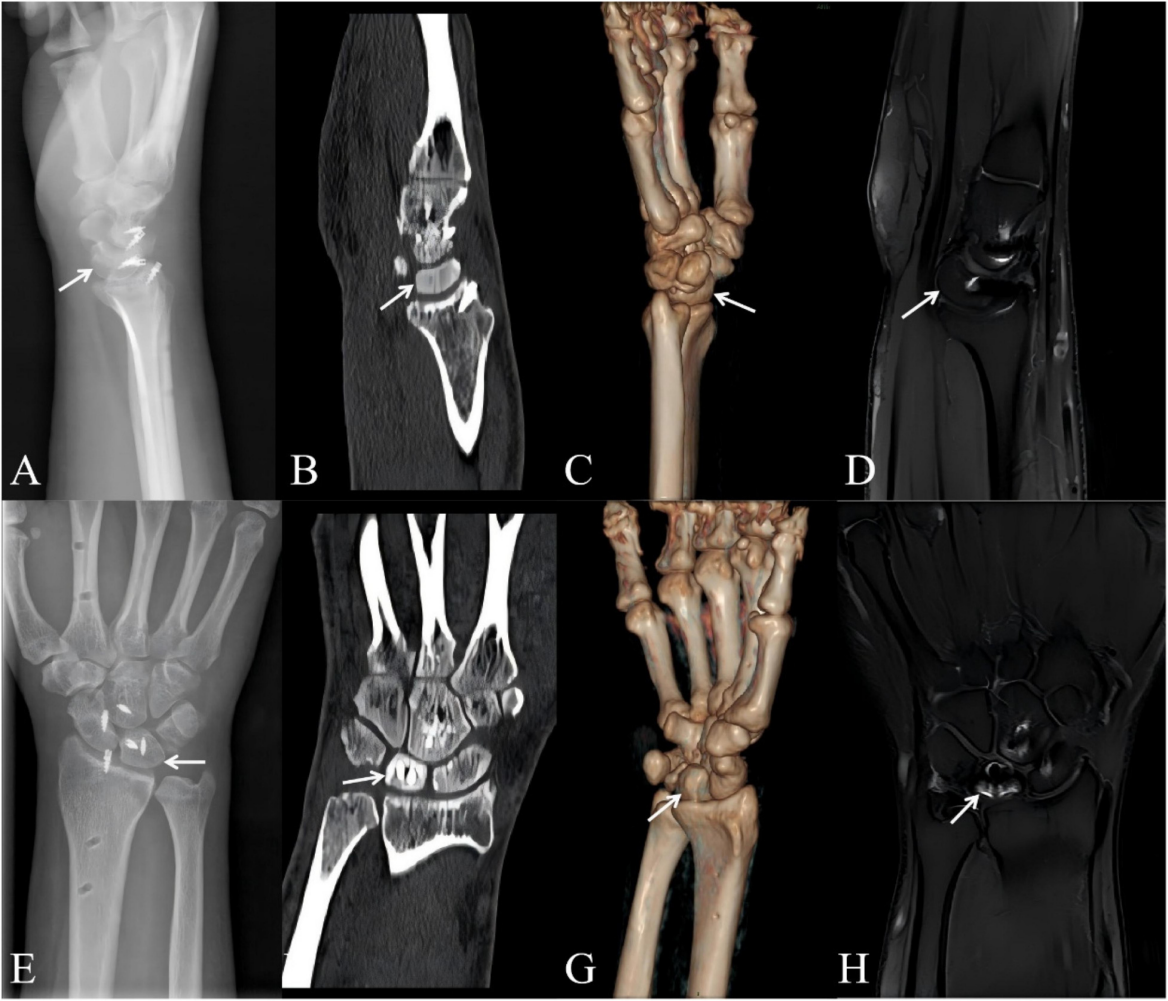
Postoperative imaging: lateral radiograph (A) and posteroanterior radiograph (E); CT scan: sagittal view (B) and coronal view (F); 3D reconstruction: lateral view (C) and posteroanterior view (G); MRI: sagittal view (D) and coronal view (H) of the right wrist. White arrows indicate lunate reduction.
